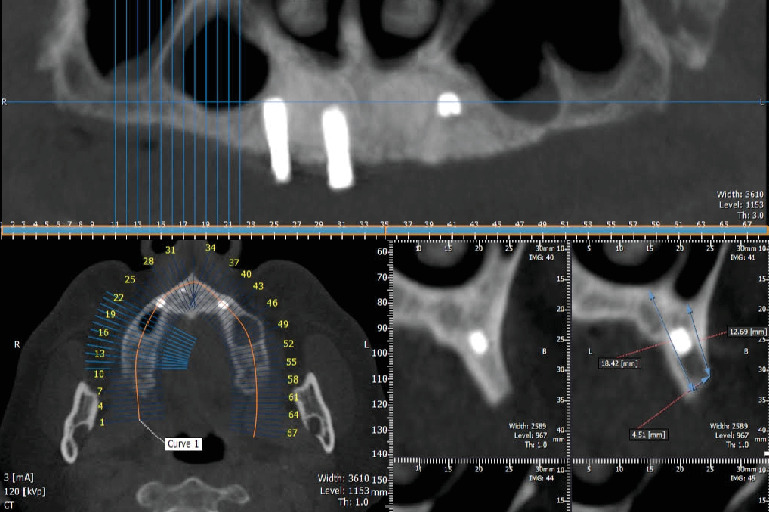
OPG and CBCT images reveal the fracture line of the implant after a month of use. Cross sections were evaluated in order to comprehend the exact position of the fracture line.
